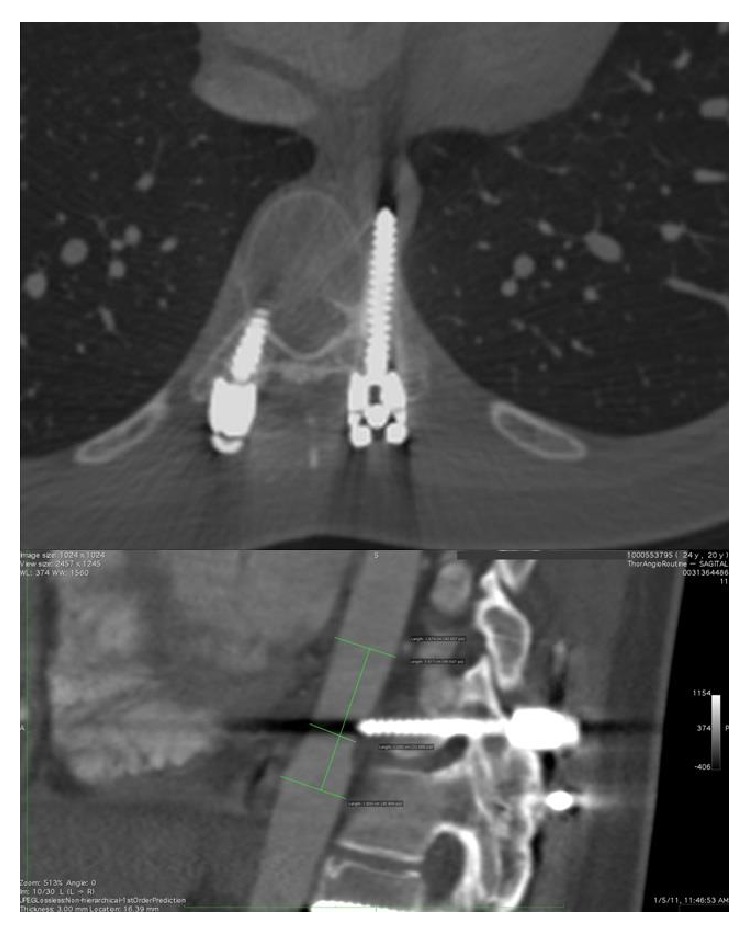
Preoperative CTA reveals the presence of the pedicle screw at the T10 level of the thoracic aorta.
